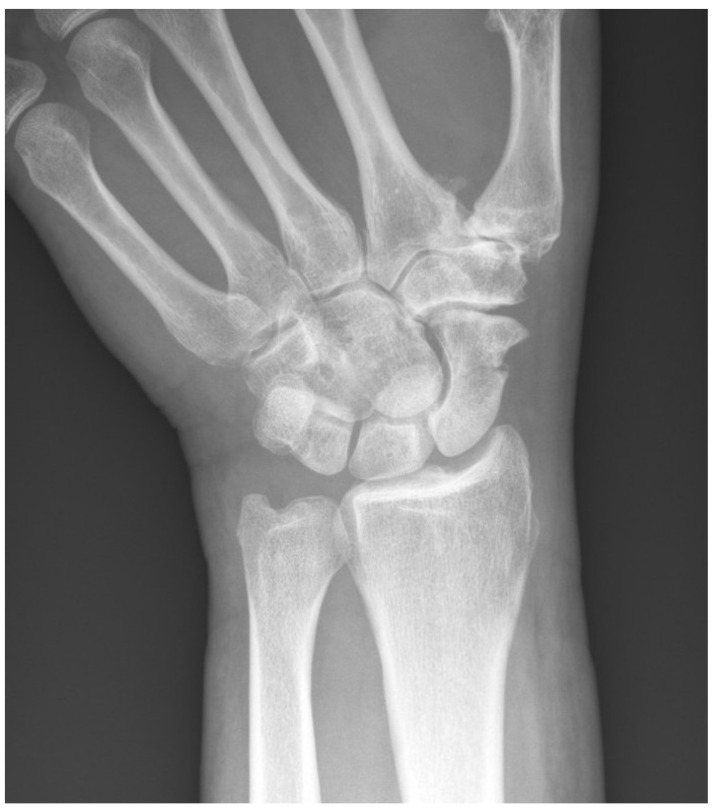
Scaphoid fracture as seen on ulnar-deviated PA scaphoid radiograph.
